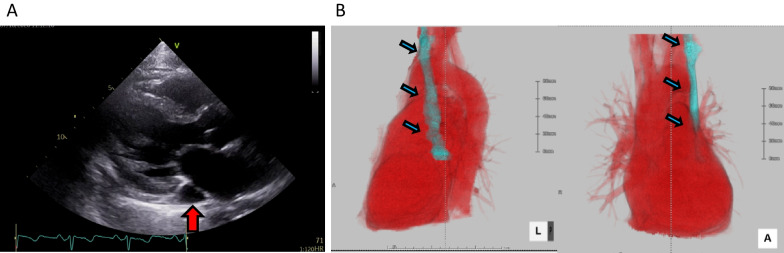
A Coronary sinus dilatation on transthoracic echocardiography. B Contrast-enhanced computed tomography: the vein draining into the right atrium through the coronary sinus at the junction of the left internal jugular and subclavian veins. Red arrow indicates enlarged coronary sinus, Blue arrows indicates persistent left superior vena cava
